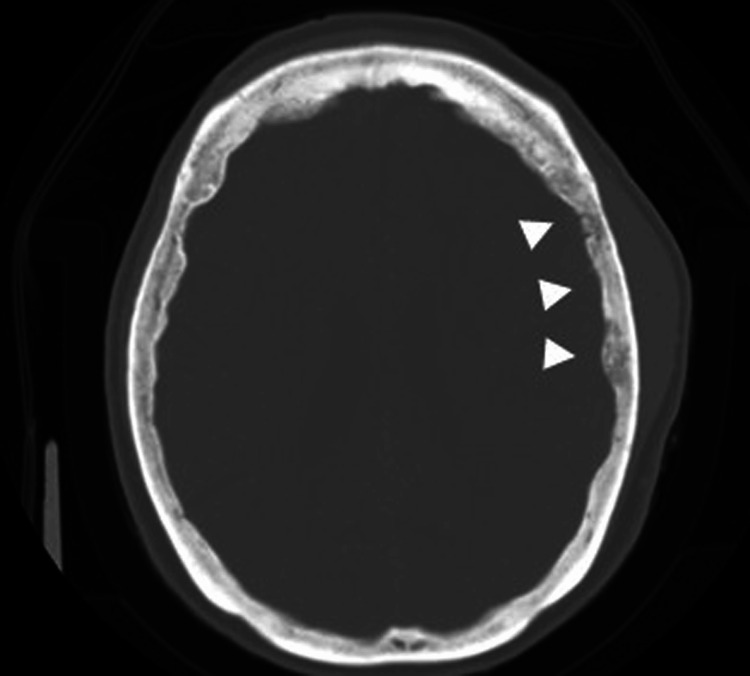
Computed tomography Computed tomography of the bone revealed no bone destruction or calcification (arrowhead).
